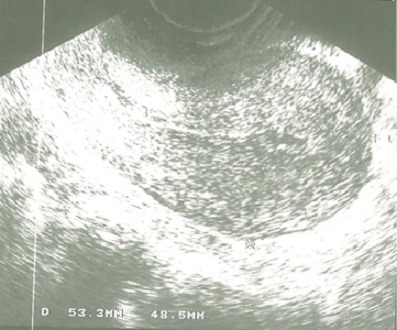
Pelvic ultrasound normal
